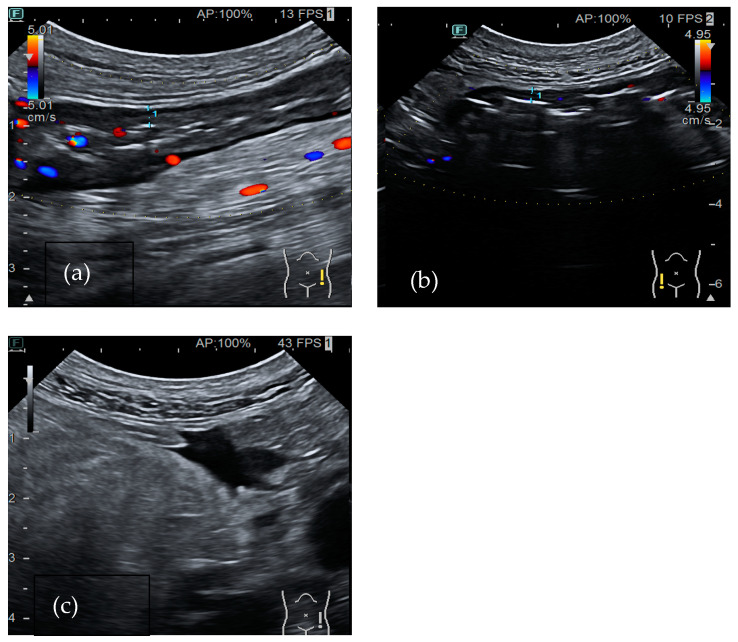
A 76-year-old-male patient was diagnosed with long-term long-term left ulcerative colitis. A bowel ultrasound was performed after the onset of bloody diarrhea during mesalamine treatment. US showed diffuse colonic wall thickening: (a) 4.7 mm in the descending colon and (b) 3.6 mm in the right colon. Active vascularization (a) with free fluid (c) was also found. The biopsies performed during colonoscopy revealed a CMV infection.
